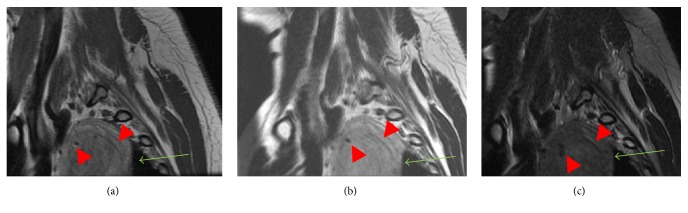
Sagittal T1 (a), T2 (b), and fat saturation T2 (c) weighted images from the cervical spine MRI show a heterogeneous, T1, and T2 hyperintense mass (green arrows in (a) and (b)) with a few internal flow voids (red arrow heads in (a), (b), and (c)) and moderate loss of signal with fat saturation (green arrow in (c)).
